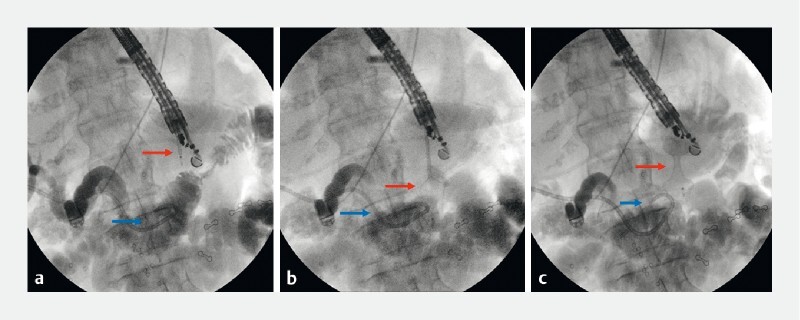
Endoscopic ultrasound (EUS)-guided jejuno-duodenal anastomosis was guided by a percutaneous transhepatic biliary drainage tube (blue arrows) connected to a water pump. A freehand EUS-guided anastomosis using a 20 mm lumen-apposing stent (red arrows) was performed under fluoroscopy control.
